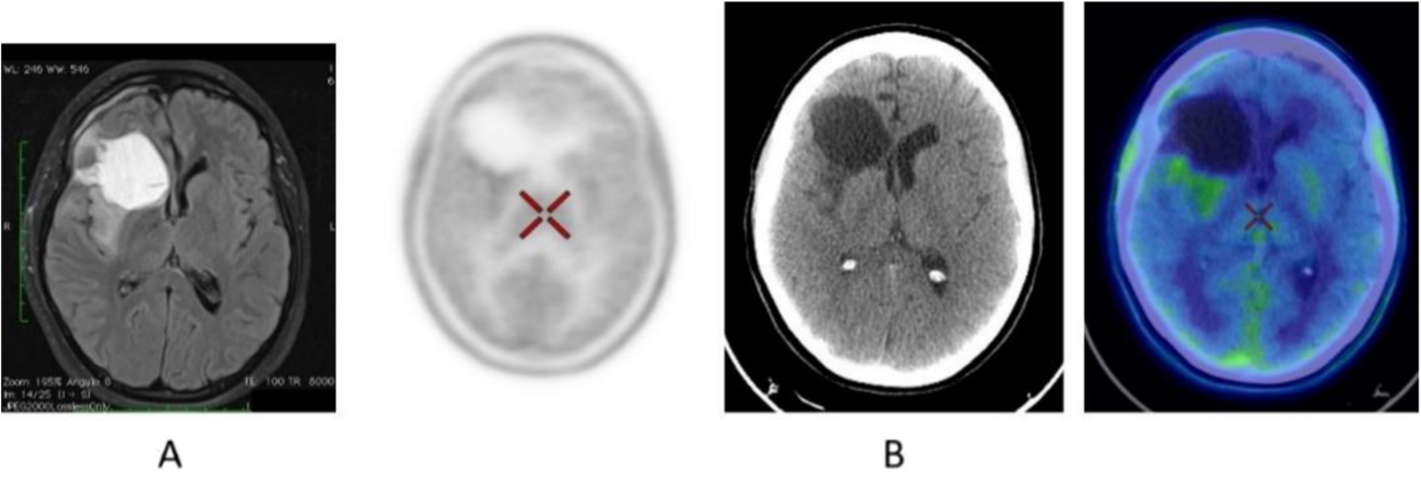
^11^C-Methionine, Glioblastoma grade 2, surgical changes. *Clinical history*: 25 y.o. female. Glioblastoma grade 2. Surgical treatment with macroscopically complete resection of right frontal glioblastoma. MRI: T2-FLAIR image shows heterogeneous hyperintensity (a) and T1 using Gd shows peripheral contrast enhancement. *PET/CT findings*: slight tracer uptake in margins of surgical field, compatible with inflammatory activity in PET/CT images (b)
